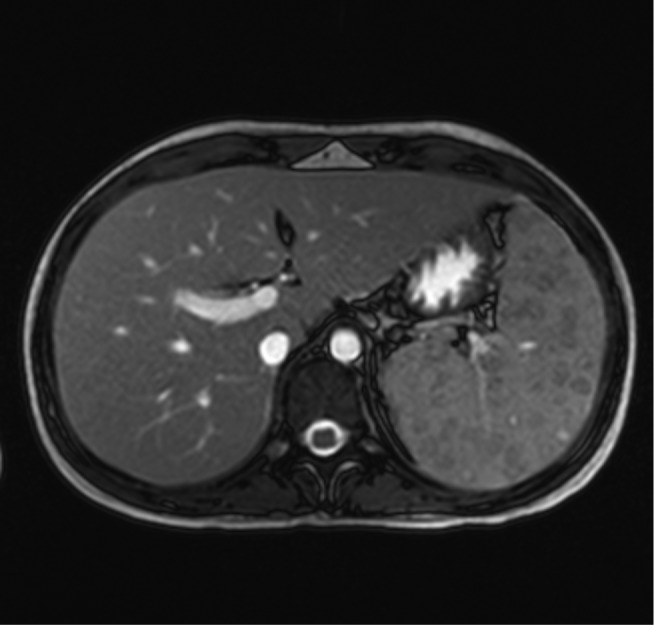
MRI of the abdomen demonstrating multiple hypogenic splenic abscesses.
